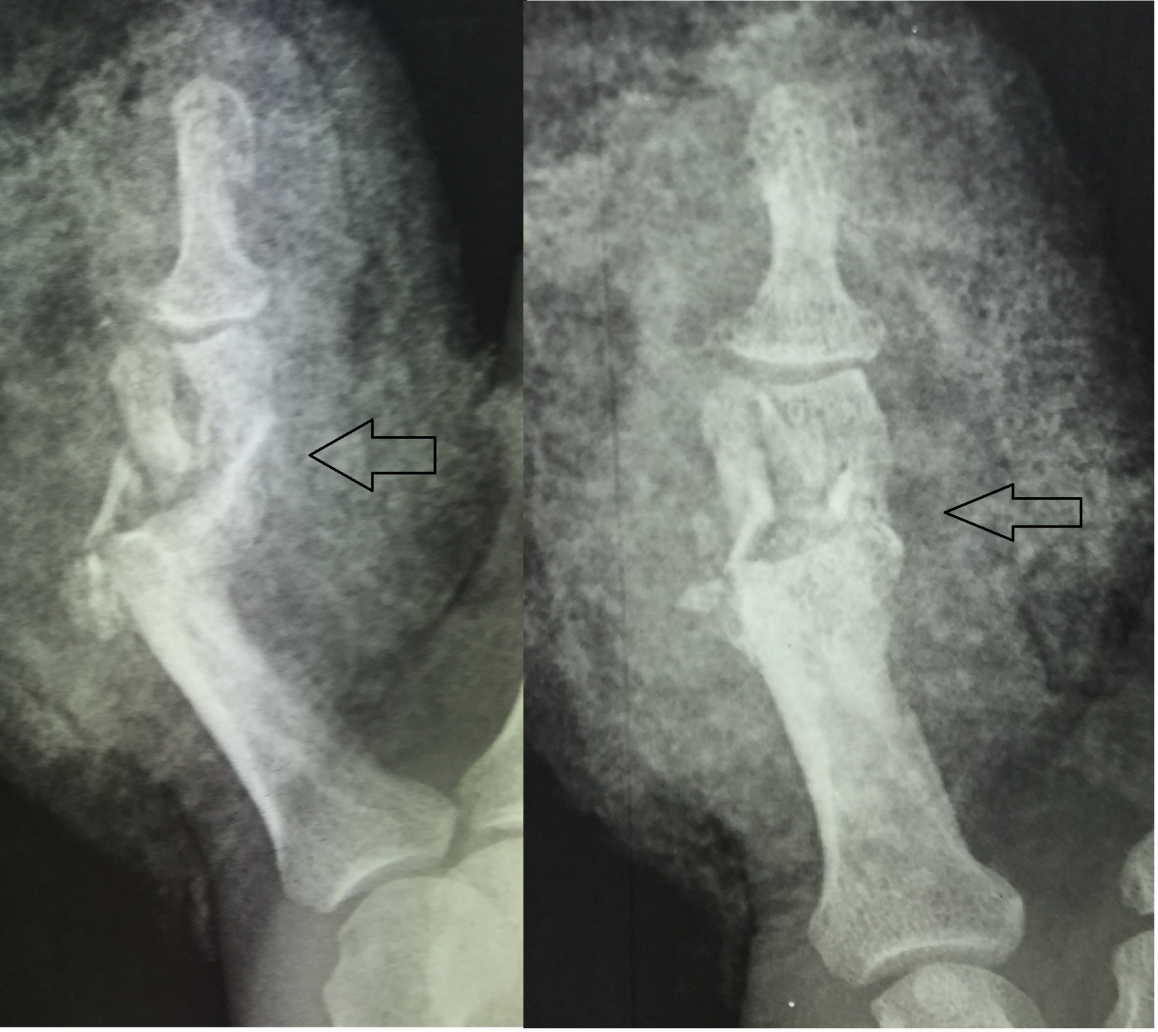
Plain radiographs: severely comminuted, intra-articular fracture of the middle phalanx
